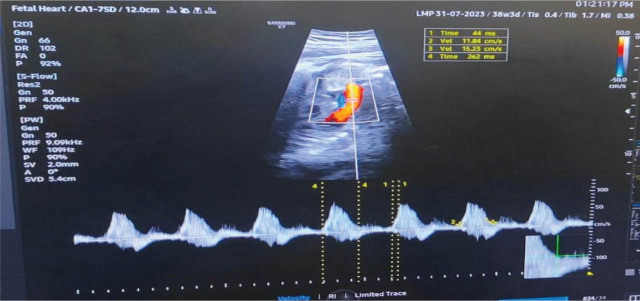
Foetal main pulmonary artery Doppler flow-trace (Ultrasound using 1–5 MHz high resolution convex matric probe, Samsung Hera i10 premium).
